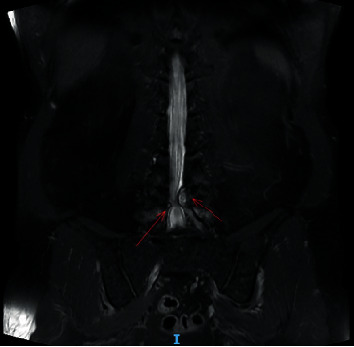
Preoperative magnetic resonance imaging (MRI) scan. This coronal T2-weighted MRI image shows cystic lesions on both sides, with each lesion arising from the right L4–5 facet joint.
